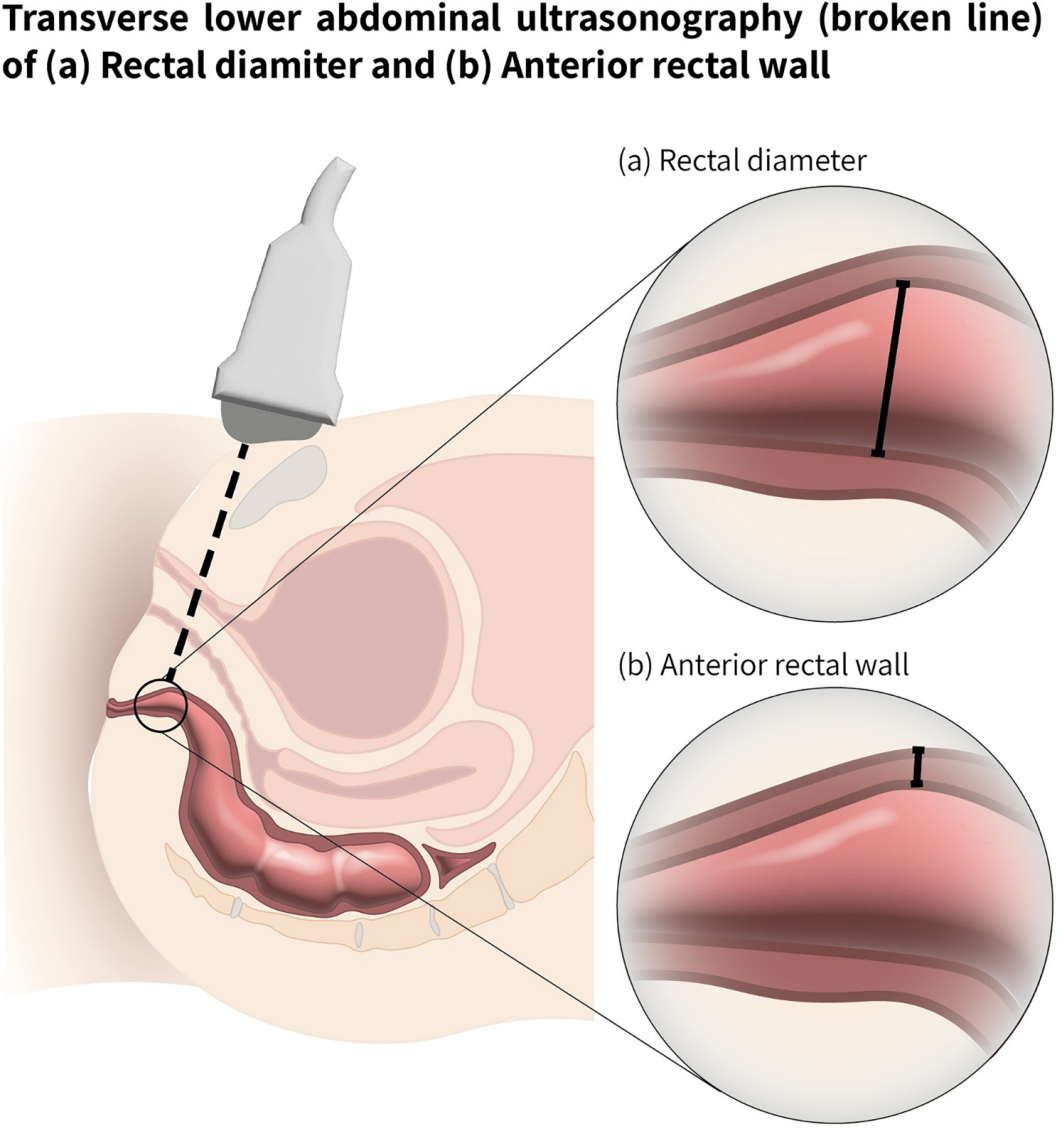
Ultrasound examination scheme for rectal measurements. Transverse lower abdominal ultrasonography, path shown with a broken line. (a) Transverse rectal diameter (TRD) shown with solid line (b) Rectal anterior wall thickness RAWT (shown with solid line).
